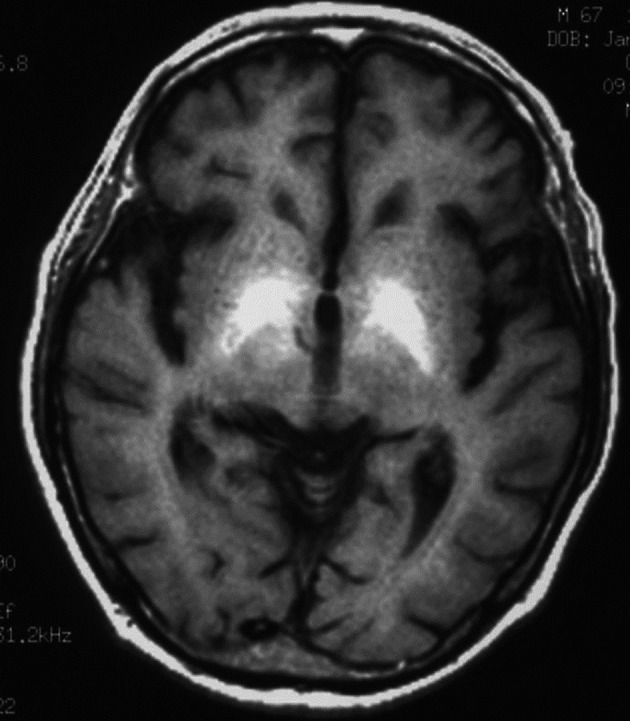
Brain MR imaging (T1WI) showed hyperintensity of basal nuclei indicating chronic hepatic encephalopathy.
